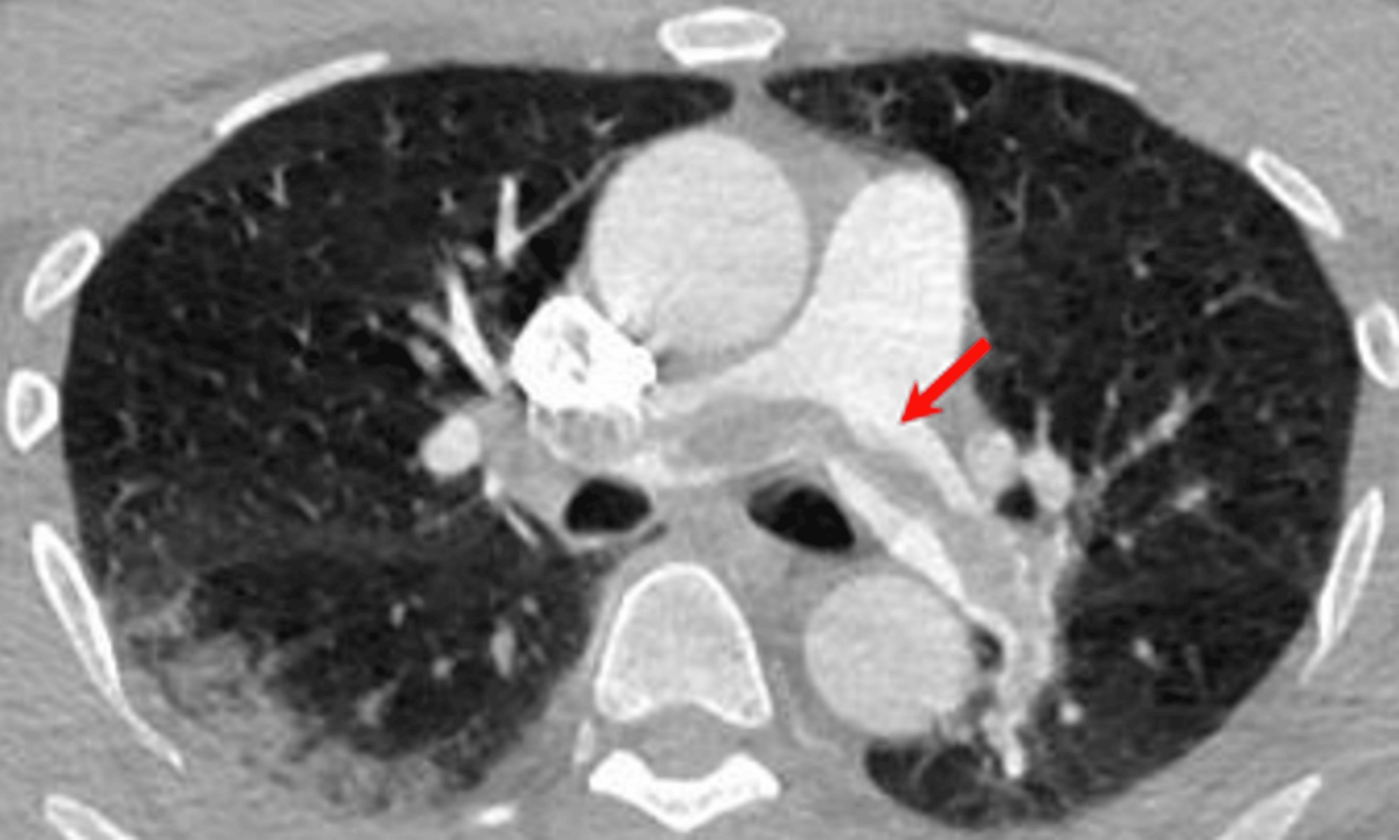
Saddle embolism in pulmonary artery seen on CTA neck which was contrast-enhanced with red arrow pointing to saddle embolism. This embolism required pulmonary embolectomy and anticoagulation to prevent likely clinical decompensation. CTA: computed tomography angiography.
